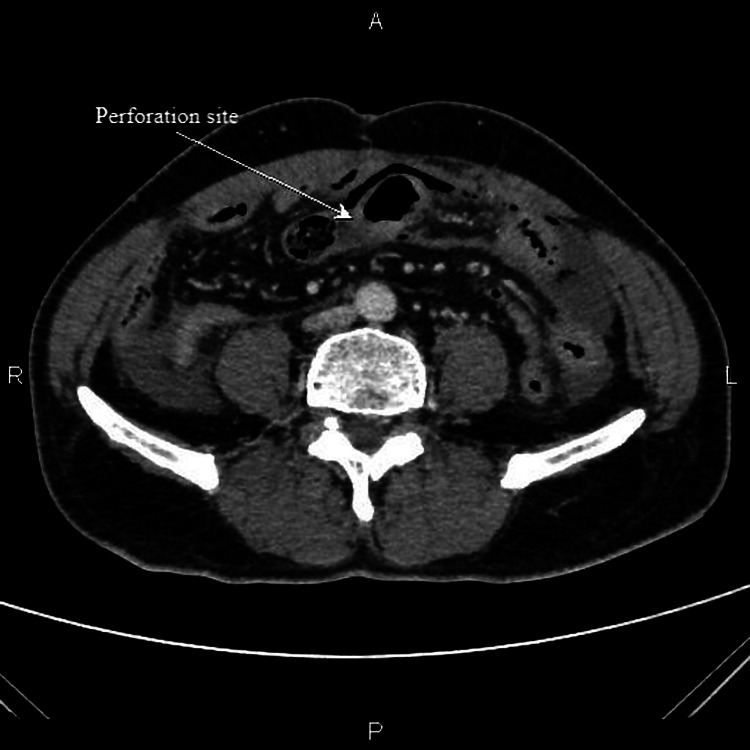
A contrast-enhanced computed tomographic scan (with both intravenous and oral contrast injected) of the abdomen in axial plane showing a defect in bowel wall near the ileocecal junction along with surrounding fat stranding and air foci (marked by arrow). P: posterior, A: anterior, R: right side
